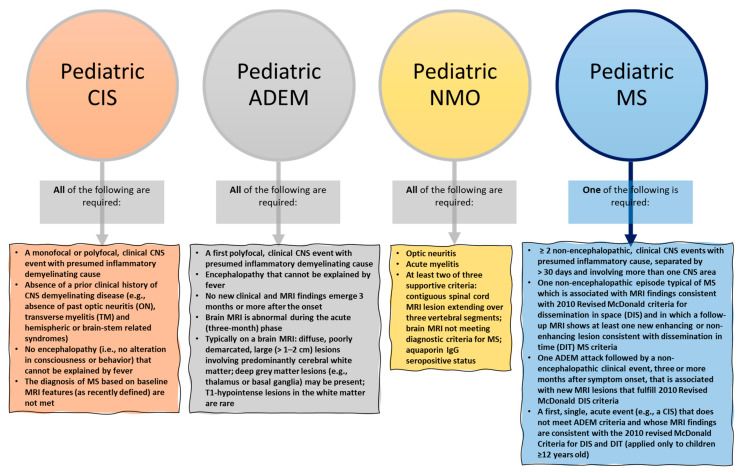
Diagnosis criteria for acquired demyelinating syndromes (ADSs). Created based on information from [12,58]. Abbreviations: ADEM—acute disseminated encephalomyelitis; CIS—clinically isolated syndrome; CNS—central nervous system; IgG—immunoglobulin G; MRI—magnetic resonance imaging; MS—multiple sclerosis; NMO—neuromyelitis optics.
